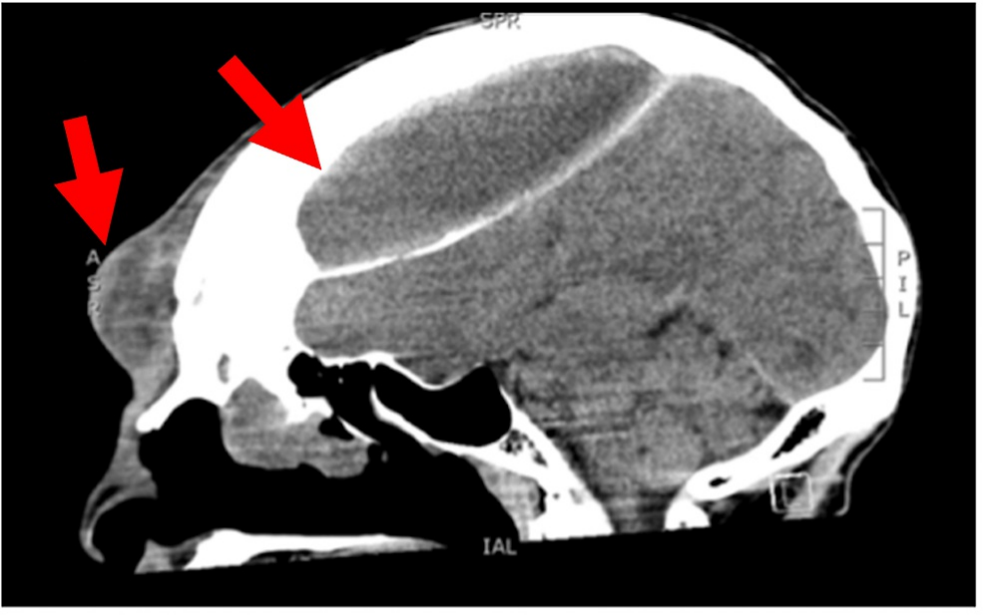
Sagittal View of CT Head and Facial Bones
